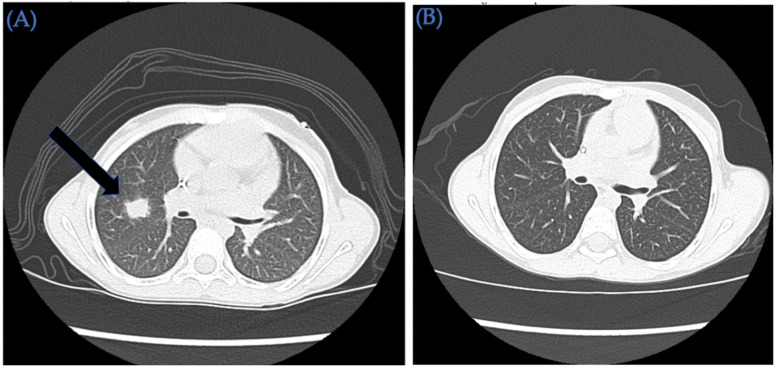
Computed tomography (CT) chest imaging of a 6-year-old Hispanic patient with relapsed AML. (A) Pre-HSCT CT chest indicates a 0.8 × 0.6 cm right upper lobe pulmonary nodule (arrow), a 0.6 cm right lower lobe lung nodule, and 0.4 cm left upper lobe pulmonary nodule. (B) Post-HSCT CT chest indicates resolving post-infectious nodules with no evidence of active infection.
